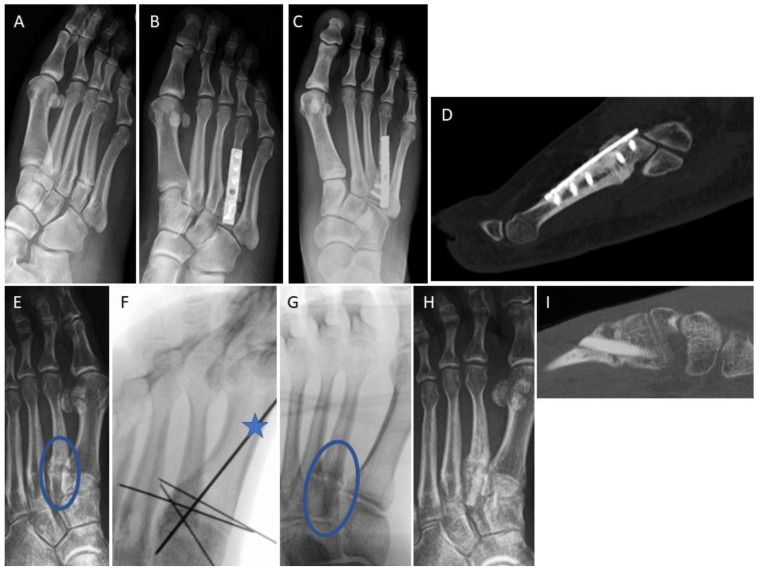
Two comparable cases with stiff non-union at the base of the lesser metatarsals. (A–D) Conventional non-union treatment. (A) A 29-year-old patient with stiff non-union at the base of the 4th metatarsal following a fracture. (B) Radiograph two weeks after conventional surgical treatment including the debridement of fibrotic/sclerotic tissue, autologous iliac crest spongiosa transplantation, and plating. (C) A weight-bearing radiograph at 3 months confirmed stable conditions. Bone healing was recorded after 8 weeks. (D) After 9 years, a CT scan was performed to plan hardware removal surgery due to persistent hardware irritation. (E–I) Treatment with a minimal invasive dorsal approach using the human allogeneic cortical bone screw. (E) A 40-year-old patient with non-union at the base of the second metatarsal (blue ellipse) after a fracture. (F) Percutaneous identification of anatomic structures using thin K-wires arranged like goal posts in the intermetatarsal space for orientation. Subsequently, a dorsal 3 cm incision was made, and a 1.6 mm K-wire (blue star) was drilled over the stiff non-union under fluoroscopic control to prepare for K-wire-guided drilling and thread cutting. (G) A single 5 mm-diameter Shark Screw^®^ (blue ellipse) was implanted and levelled with a bone saw at the dorsal metatarsal cortex. Fluoroscopy confirmed the accurate transplant position. (H) The patient’s recovery progressed well, allowing full weight bearing after 4 weeks, and clinical recovery was achieved. (I) A 6 week CT scan showed direct bone contact and the bridging of the former non-union. By this time, the non-union was clinically consolidated, and the patient was pain-free.
